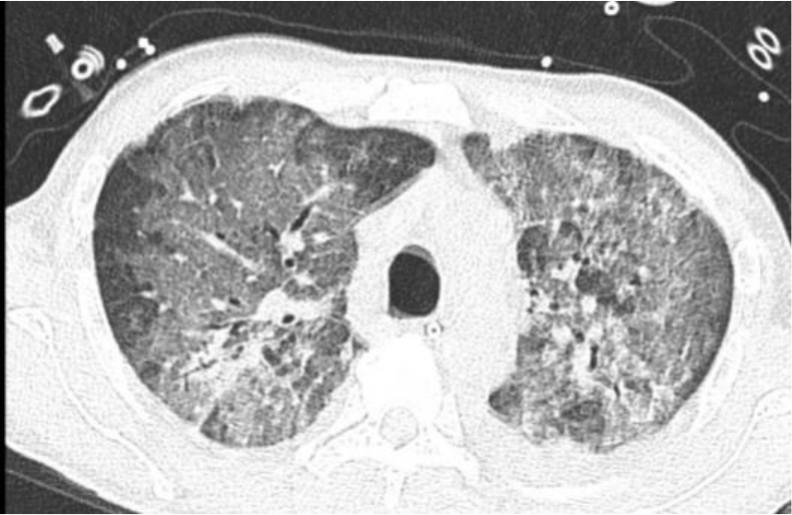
Case #2: CT image showing diffuse ground-glass changes.
